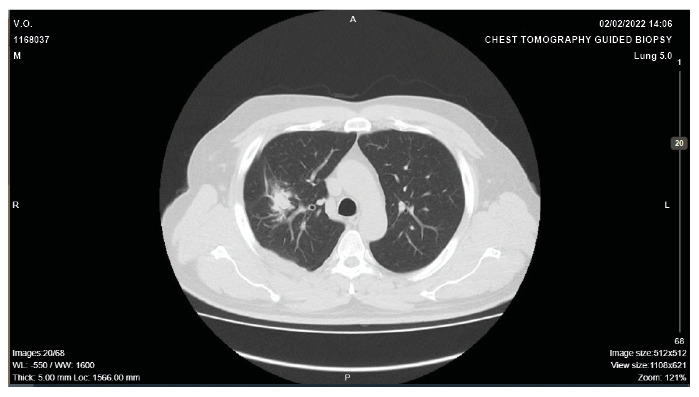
Chest computed tomography with a mediastinal window showing a lesion in the right hemithorax.
